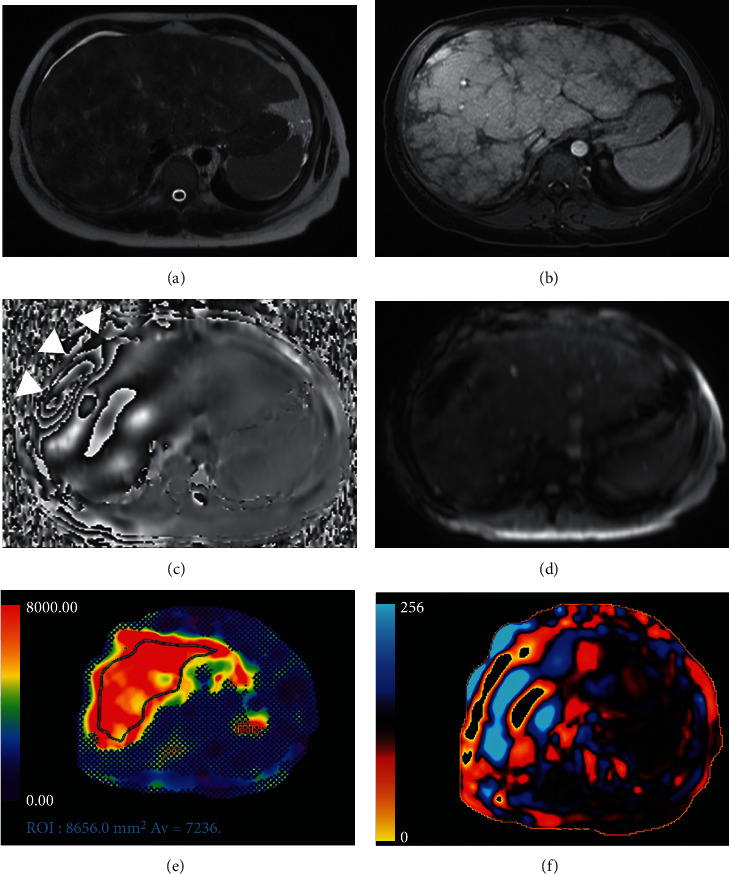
MRE images of a 38-year old female patient with NAFLD. (a) axial single-shot spin echo T2-weighted image shows the severe liver tissue alterations. (b) shows axial GRE T1-weighted fat-saturated image after contrast medium administration (gatoxetic acid), during hepatobiliary phase. (c) Axial GRE MRE image where it is possible to appreciate the propagation effect generate from the passive pneumatic driver (arrowheads). (d) Magnitude image. (e) Color elastogram with 95% confidence map. Free hand manual ROI is drawn on liver parenchyma on a large area of the liver that is not covered by the 95% confidence map shows an average stiffness value > 5 kPa, corresponding to a stage 4 fibrosis. (f) Axial wave image showing thick and irregular waves expression of hepatic fibrosis. MRE: magnetic resonance elastography; NAFLD: nonalcoholic fatty liver disease; GRE: gradient echo; ROI: region of interest.
